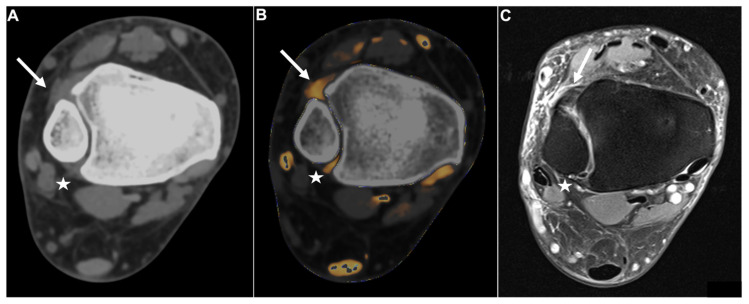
Intact DTFS. Standard axial unenhanced grayscale CT (A), color-coded collagen reconstructions (B), and proton density-weighted unenhanced MRI series with fat saturation (C) of a patient with an ankle sprain. No diagnostic assessment of the DTFS is possible in the grayscale images. After collagen mapping, the ATIFL (arrow) and PTIFL (star) demonstrate strong collagen signals, indicating that they are not injured. MRI confirmed the integrity of both ligaments. Abbreviations: DTFS, distal tibiofibular syndesmosis. ATIFL, anterior tibiofibular ligament. PTIFL, posterior tibiofibular ligament.
